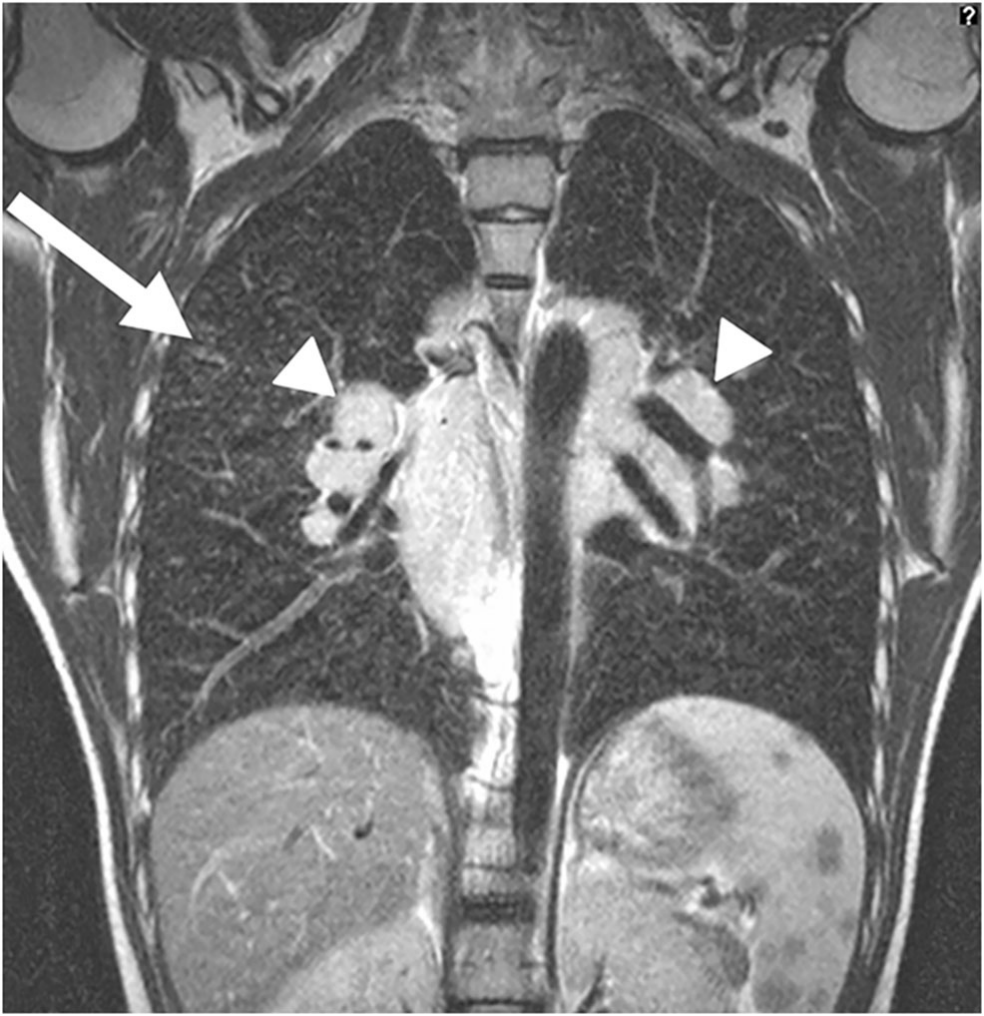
Sarcoidosis Stage II in a 15-year-old girl. Lung MR (coronal T2-weighted turbo spin-echo image, parameters in Table 2) shows many small granulomas, especially in the right lung (*arrow*). This finding, in conjunction with hilar lymph node enlargement (*arrowheads*), is typical for sarcoidosis. A central signal reduction in the lymph nodes, which we could not observe in our patient, is also known to be characteristic. Please also note the low-signal granulomas in the spleen
